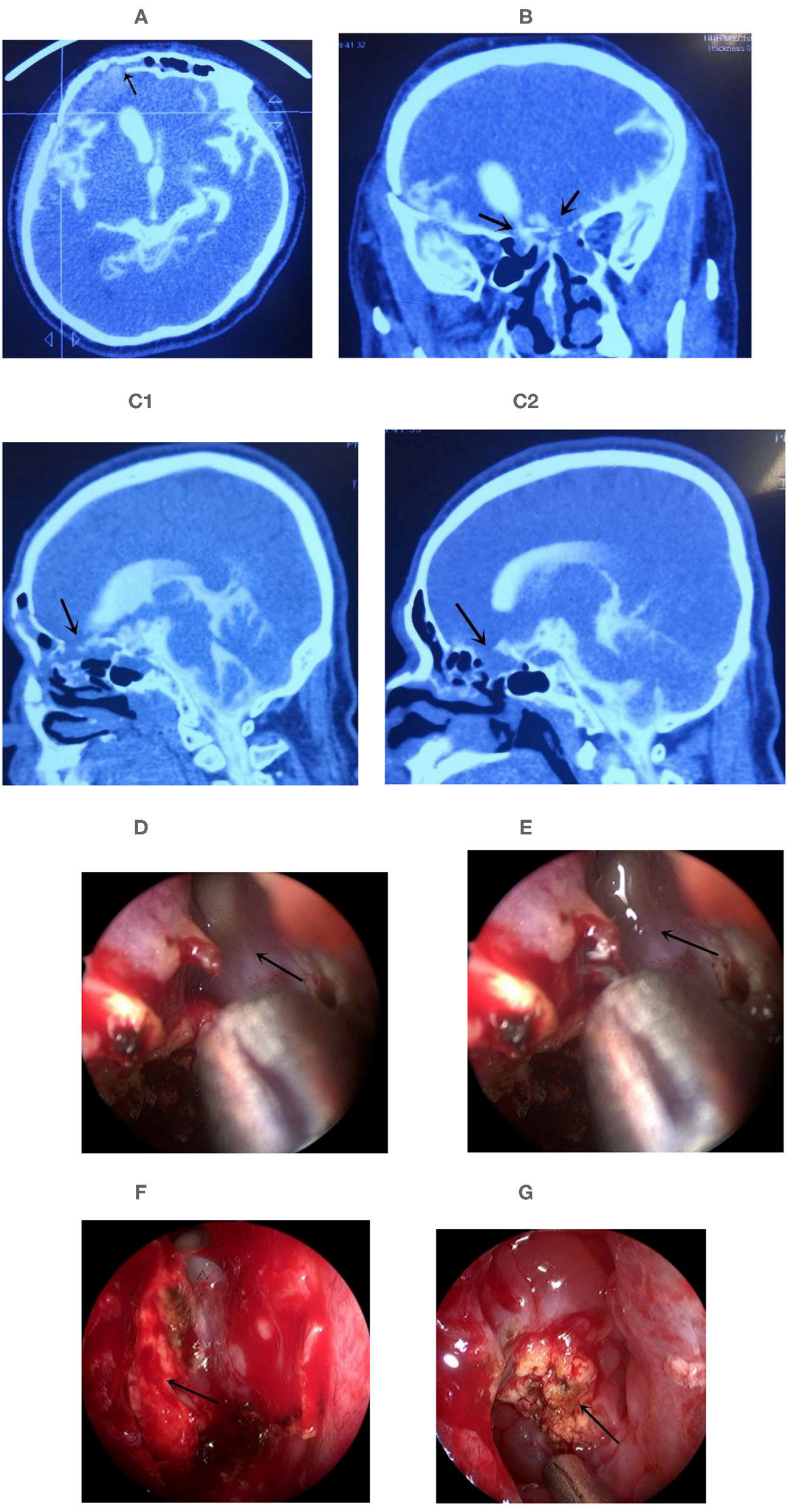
Multiple leaks were repaired by transnasal endoscopy combined with the epidural approach. (A–C) The CTC examination located the leaks before surgery. (A) The leak was in the right frontal sinus; (B) The leak was located in the both ethmoid sinus roofs of the coronal CT. (C1) Sagital CT of right ethmoid, (C2) Sagital CT of left ethmoid. Arrow show the suspected leak. (D) No CSF leak in the right frontal sinus, arrow show the orifice of frontal sinus. (E) CSF leak was found in the right frontal sinus when intraoperative injection of 40 mL normal saline through lumbar drainage to expose the leak. Arrow show the CSF in the orifice of frontal sinus. (F) Encephaloceles in the right ethmoid sinus roof leakage, arrow show the encephaloceles, triangle indicated the orifice of right frontal sinus; (G) Encephaloceles in the left ethmoid sinus roof leakage, arrow show the encephaloceles (Supplementary Video 2).
